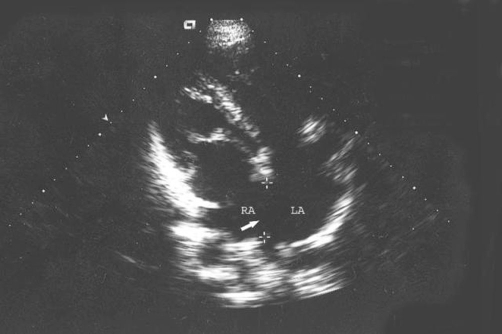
Preoperative echocardiographic evaluation shows atrial septal defect (arrow; between calipers; RA: right atrium, LA: left atrium).
